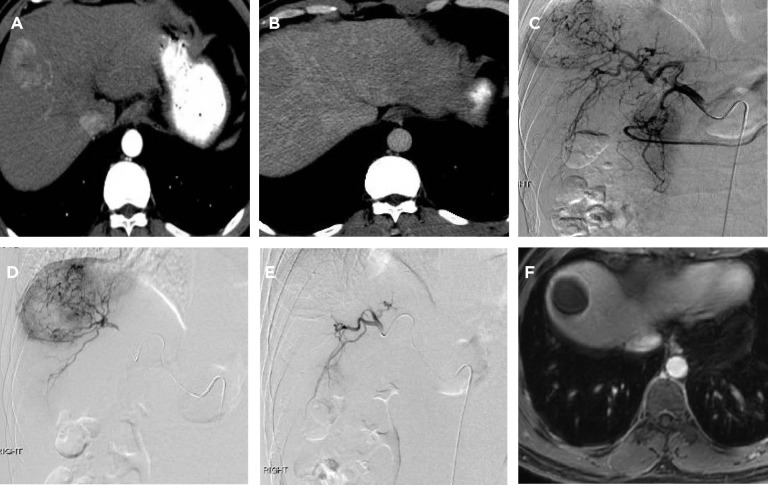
THA’s scans. (A) Arterial-phase CT scan shows the 4 cm mass in the liver; (B) Delayedphase CT scan of the liver shows the "washout" of contrast typical for hepatocellular carcinoma; (C) Right hepatic arteriogram shows the tumor vascularity in the dome of the liver; (D) Selective segmental right hepatic arteriogram shows the tumor vascularity; (E) Postembolization right hepatic arteriogram shows no further filling of the tumor; (F) Contrast-enhanced arterial-phase MR imaging 6 weeks after DEB-TACE shows no residual tumor vascularity.
